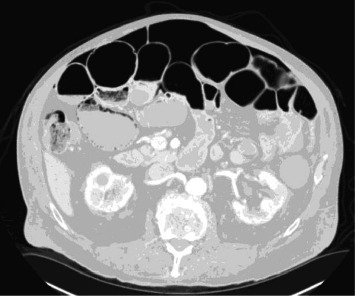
Low-dose CT images for lung nodule surveillance protocol demonstrating massive free air underneath the diaphragm.
